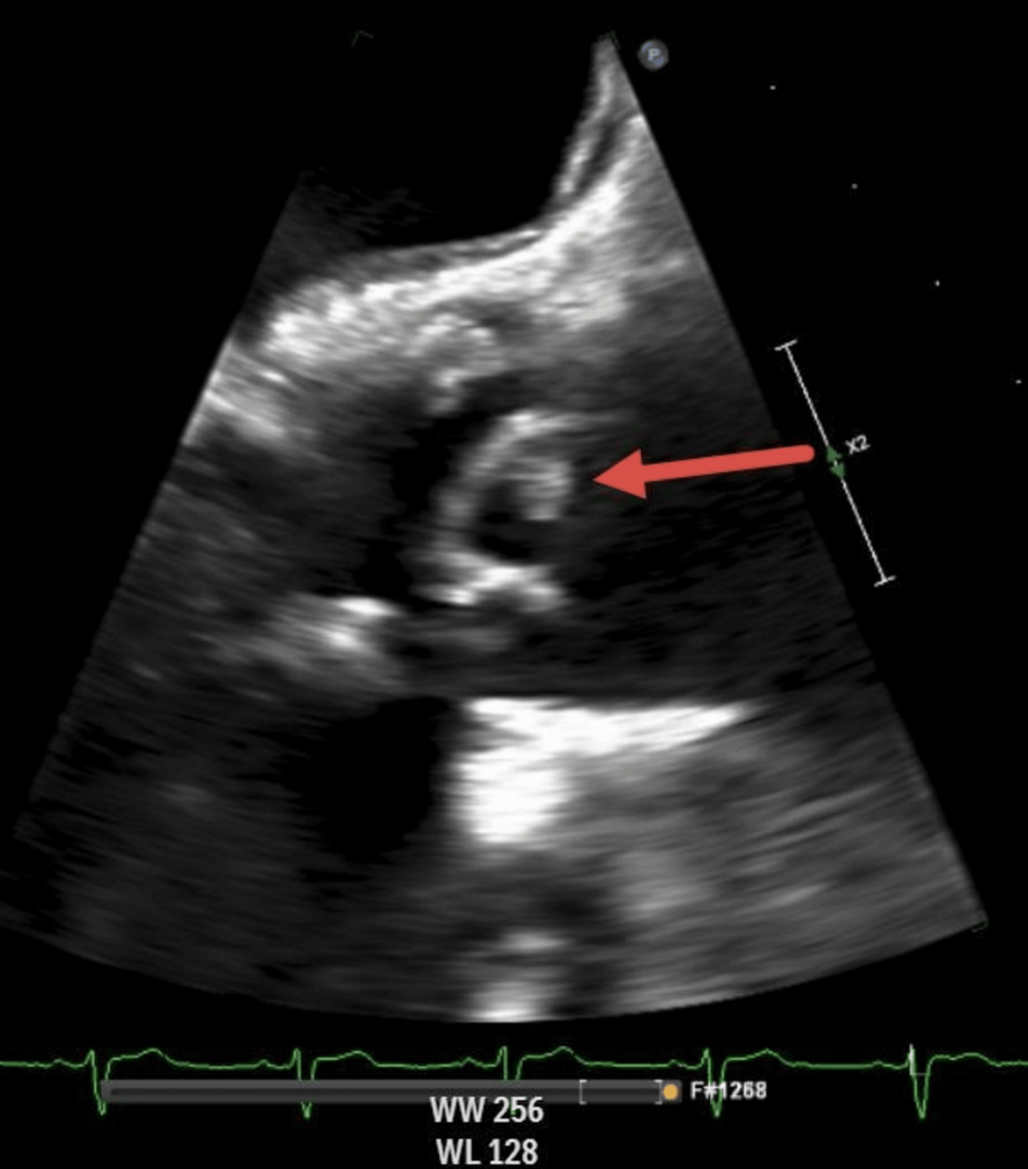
Transesophageal echocardiogram Longitudinal view of approximately 0.5 cm vegetation (red arrow). Mobile echodensity on one of the leaflets of the bioprosthetic aortic valve is concerning for endocarditis.
